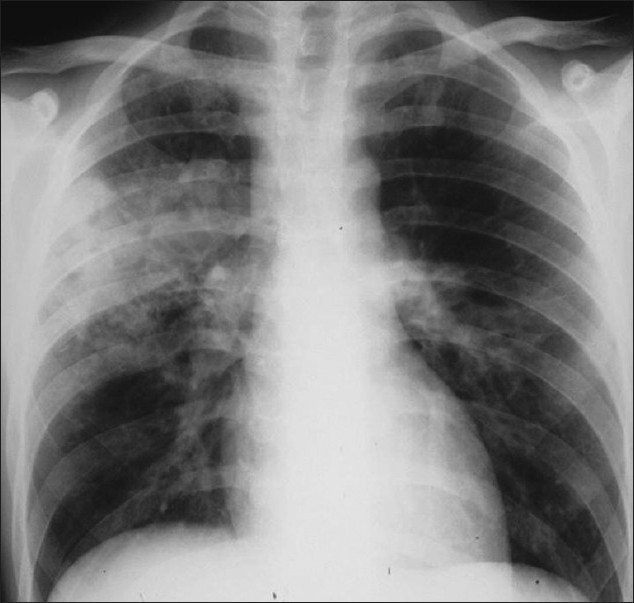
“Primary” *Mycobacterium tuberculosis*. Chest X-ray shows right upper lobe and left midzone consolidation and adenopathy. Note lack of cavitation in this patient with a low CD4 count
